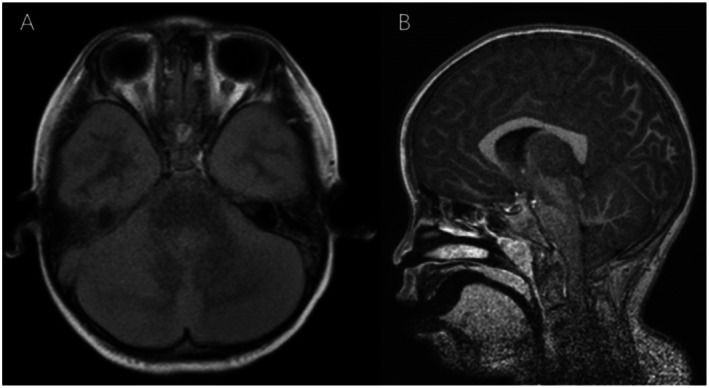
Cranial magnetic resonance imaging. (A) Magnetic resonance imaging revealed widespread shallow cerebral sulci, indistinct gray‐white matter differentiation, generalized abnormal signal intensity in the cerebral and cerebellar parenchyma, with low signal on T1‐weighted images, high signal on T2‐weighted images, high signal on fluid attenuated inversion recovery images, and high signal on diffusion‐weighted imaging; (B) There was displacement of the cerebellar tonsils laterally located below the foramen magnum and becoming pointed. The lowest point is at the level of the C3 vertebral body.
